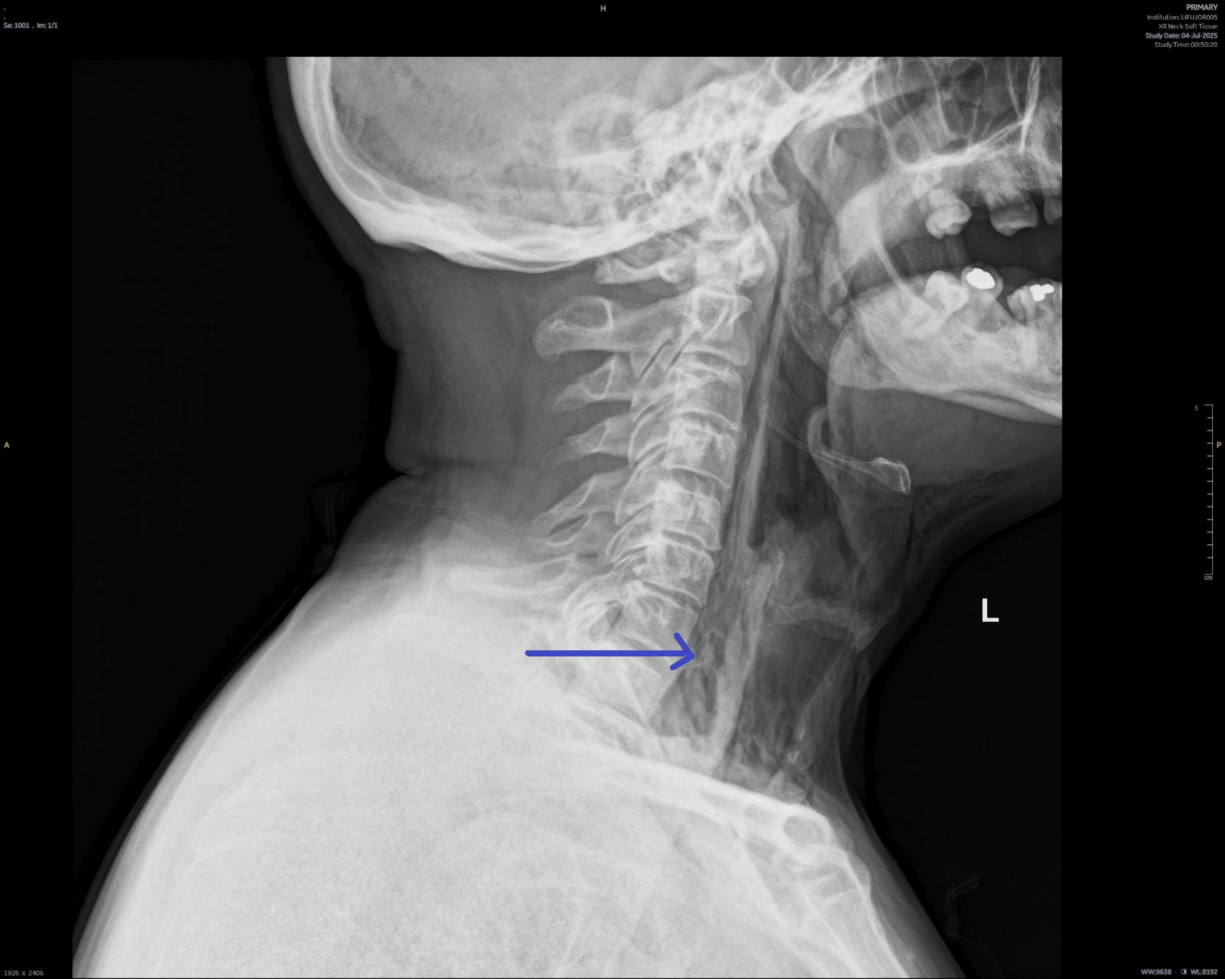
CT of the neck with contrast Pneumomediastinum noted mainly posteriorly along with soft tissue neck emphysema. Appearances are compatible with esophageal rupture.
